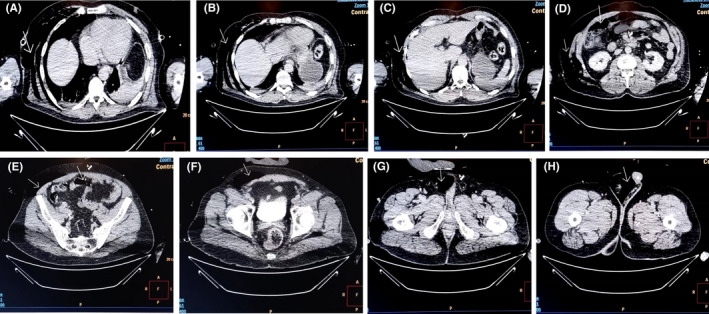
(A–H) Axial CT images demonstrating surgical emphysema (white arrows) overlying the anterolateral and posterior soft tissues of the right chest extending down to the scrotum
